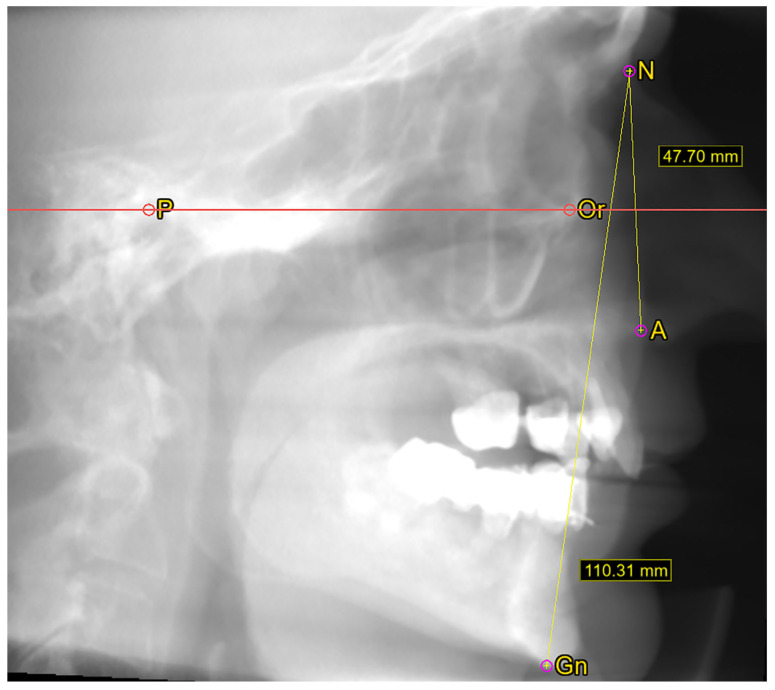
Lateral cephalometric radiograph. P—porion; Or—orbitale; N—nasion; ANS—anterior nasal spine; Gn—gnathion. The case was aligned with the Frankfurt plane positioned horizontally. Measurements were taken for both facial height and midface height.
